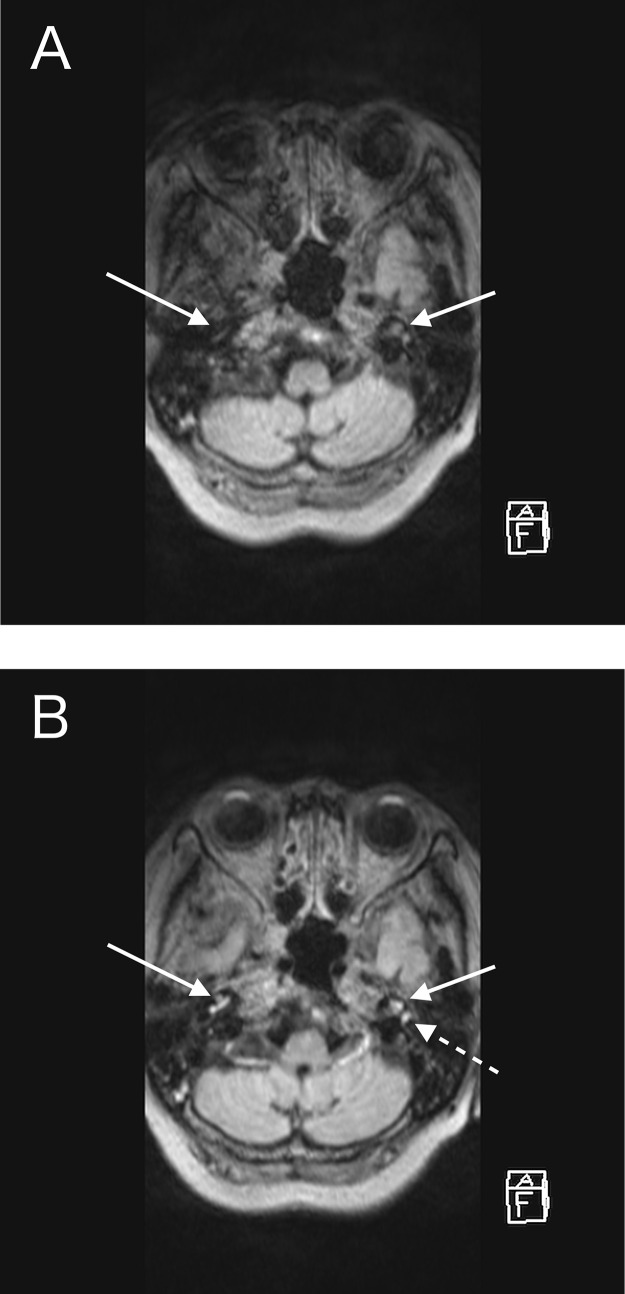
(A) Nonenhanced FLAIR showed isointensity of the chochleae and semicircular canals (arrows). (B) CE-FLAIR images showed bilateral enhancement of the cochlea (arrows) and semicircular canals (dashed arrow).
